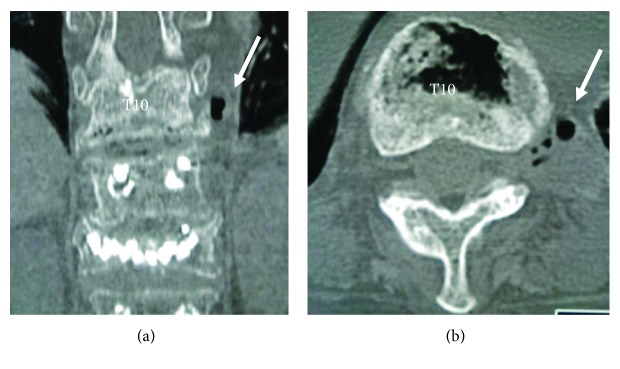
Preoperative coronal (a) and axial (b) computed tomographies of the thoracic spine obtained in the supine position, more clearly showing the gas and fluid collections (arrows) just below the left 10th rib head in (a) and the T10-T11 extraforaminal zone in (b).
